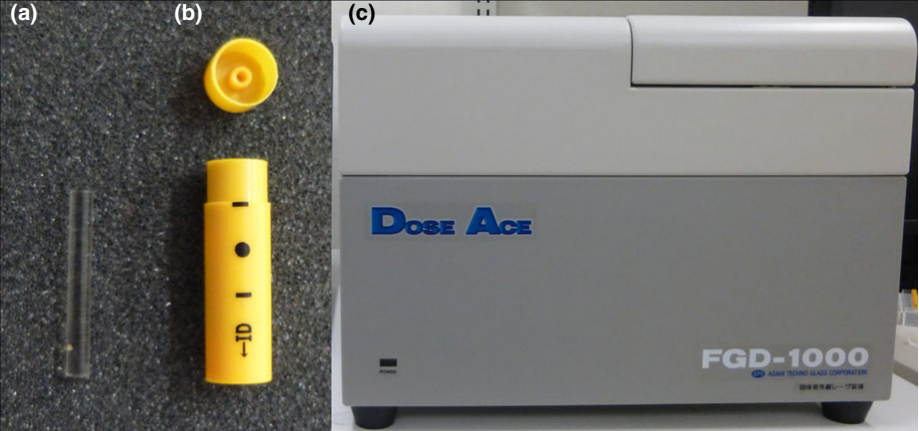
GD352M glass dosimeters (left), a tin filter (center), and a FDG 1000 reader (right).
